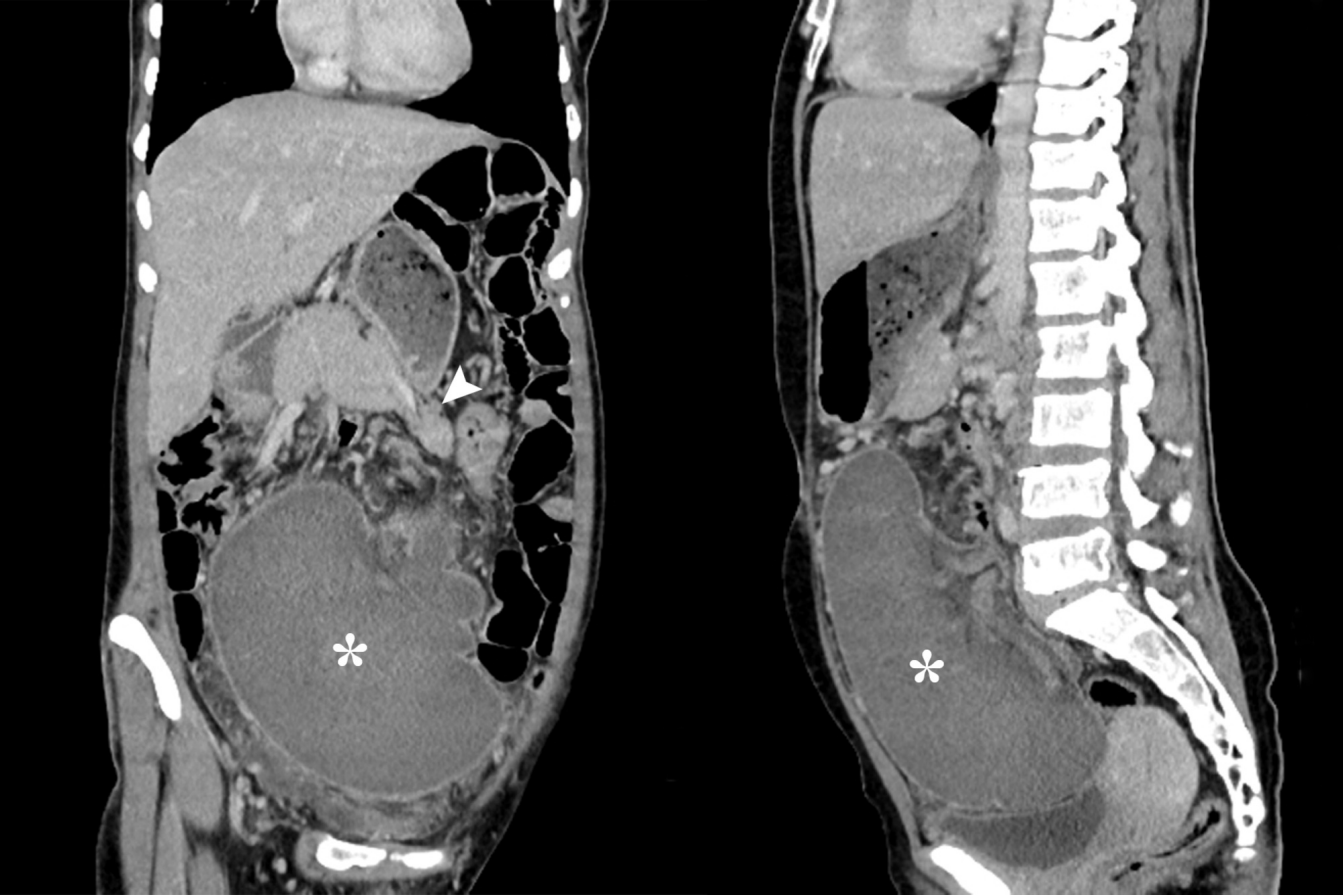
Coronal and sagittal computed tomography images. Reformatted images showing abnormal position of the spleen (asterisk). Also seen is the pancreatic tail being pulled along with the vascular pedicle (arrowhead).
